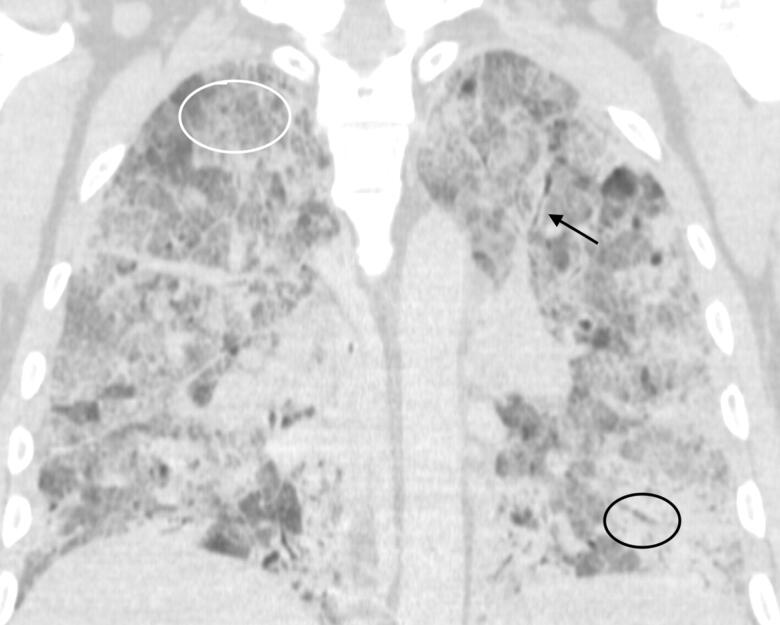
Postmortem CT (PMCT) coronal chest: one 54-year-old male with fever and cough with positive test for SARS-CoV-2. Pulmonary findings of COVID-19 in the deceased are shown with bronchial wall thickening (black arrow), air bronchograms as depicted as visible air within the bronchi surrounded by dense consolidated lung parenchyma (black circle) and a background of ground glass opacification as hazy appearance without underlying obscuration of the bronchi and vessels (white circle).
